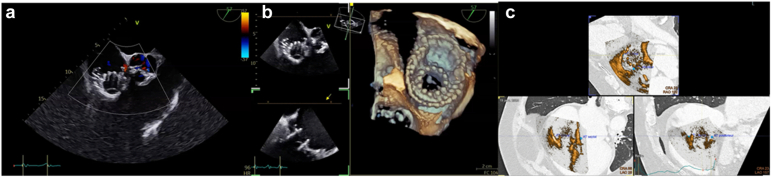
Final Evaluation. (a) Final device deployment and the degree of residual TR are assessed using Color Doppler imaging, (b) three-dimensional echocardiography with and without multiplanar reconstruction (MPR), and (c) echo-CT fusion imaging Abbreviations: TR, tricuspid regurgitation; CT, computed tomography.
